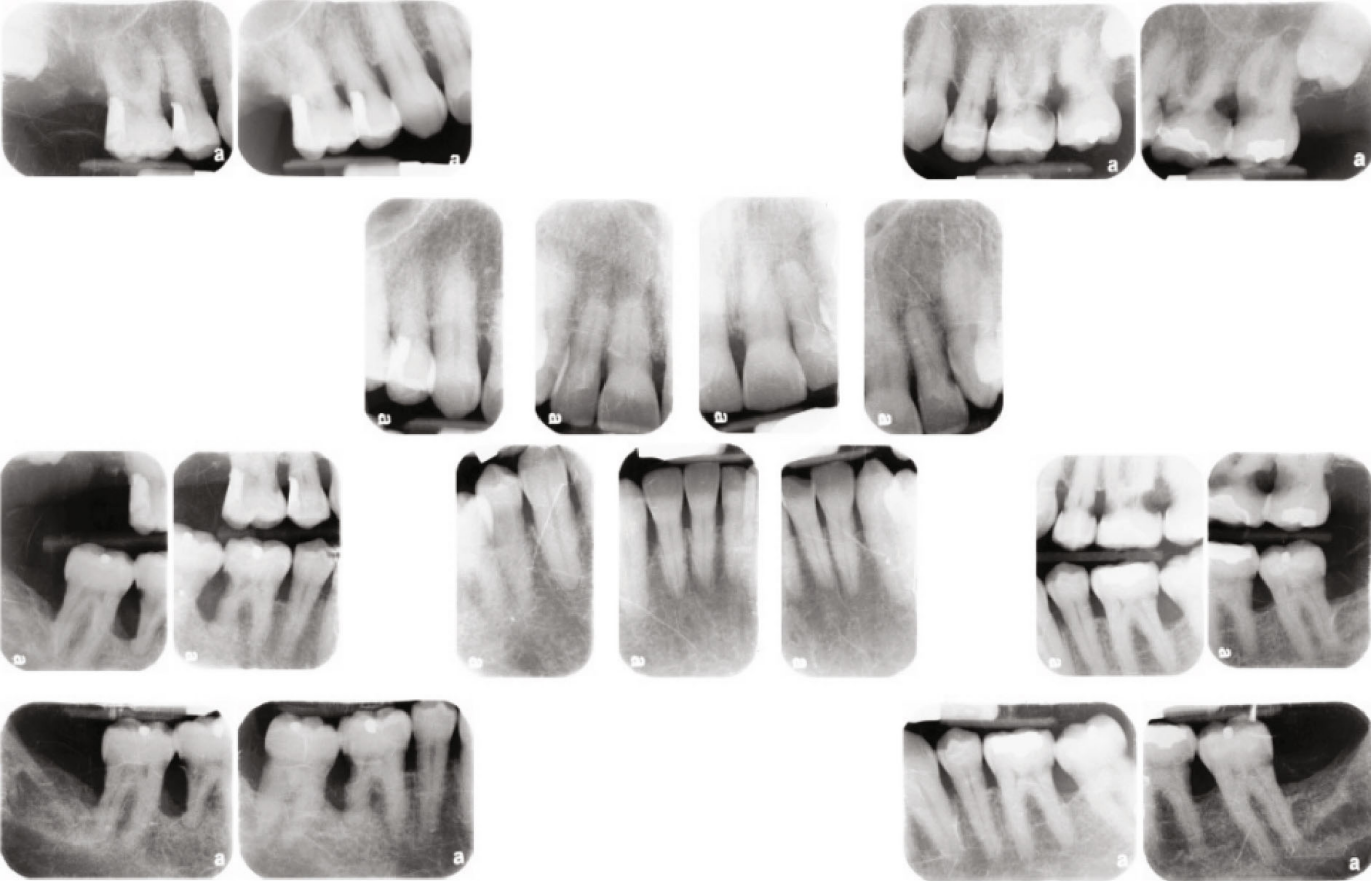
Full-mouth radiographic survey (FMRS) taken at the periodontal office, December 1, 2009.
